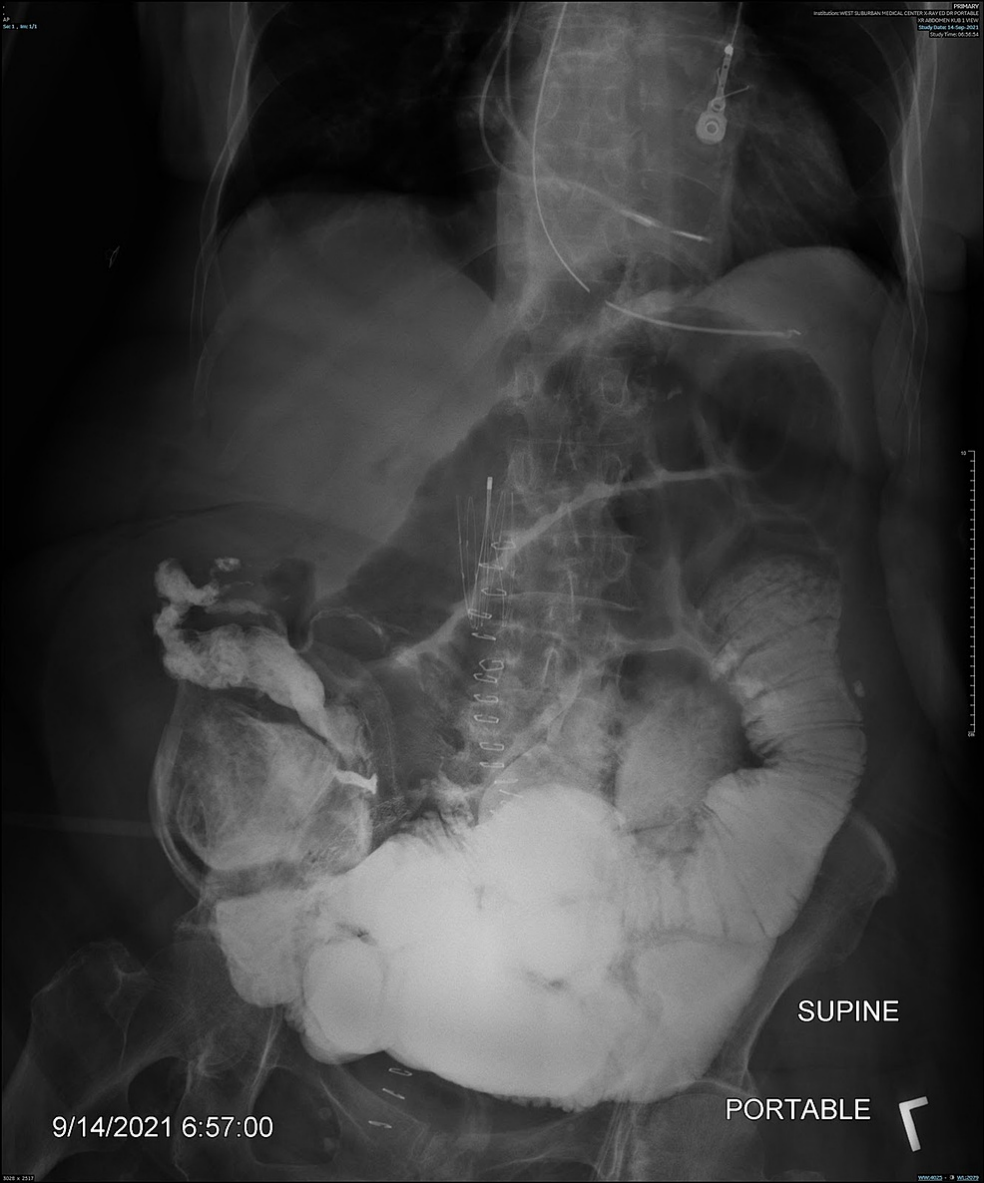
Abdominal radiograph (KUB) obtained 13 days after the first exploratory laparotomy, showing loops of the small bowel dilated by gas, fluid, and contrast, indicative of persistent SBO. KUB, kidney-ureter-bladder; SBO, small bowel obstruction
